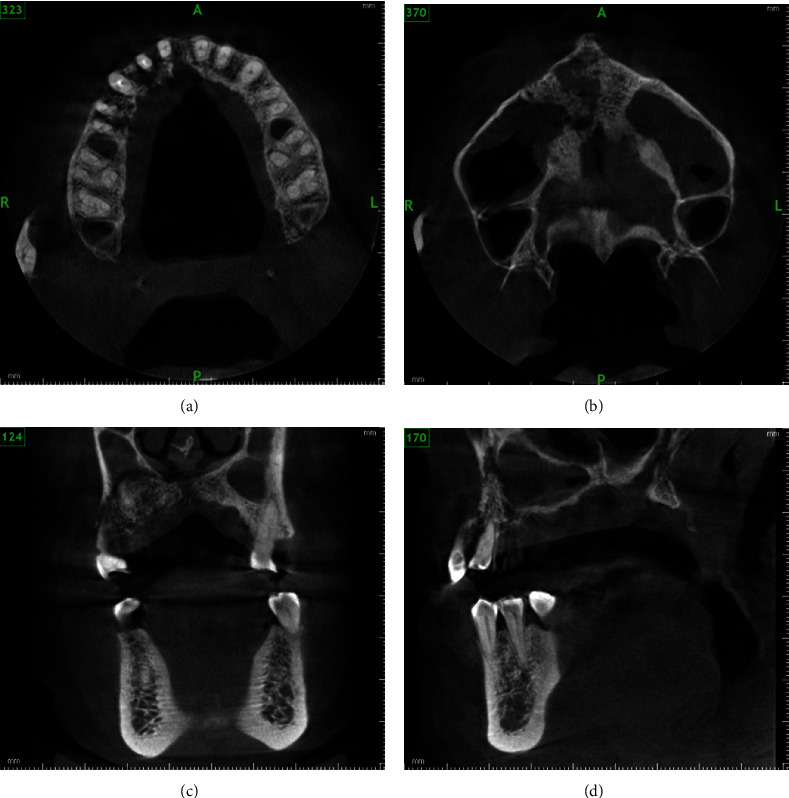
CBCT images of case 3 showing (a, b) axial views with osteolytic changes in the alveolus and palate with perforating sinus floor as seen in coronal views and sagittal views (c, d).
